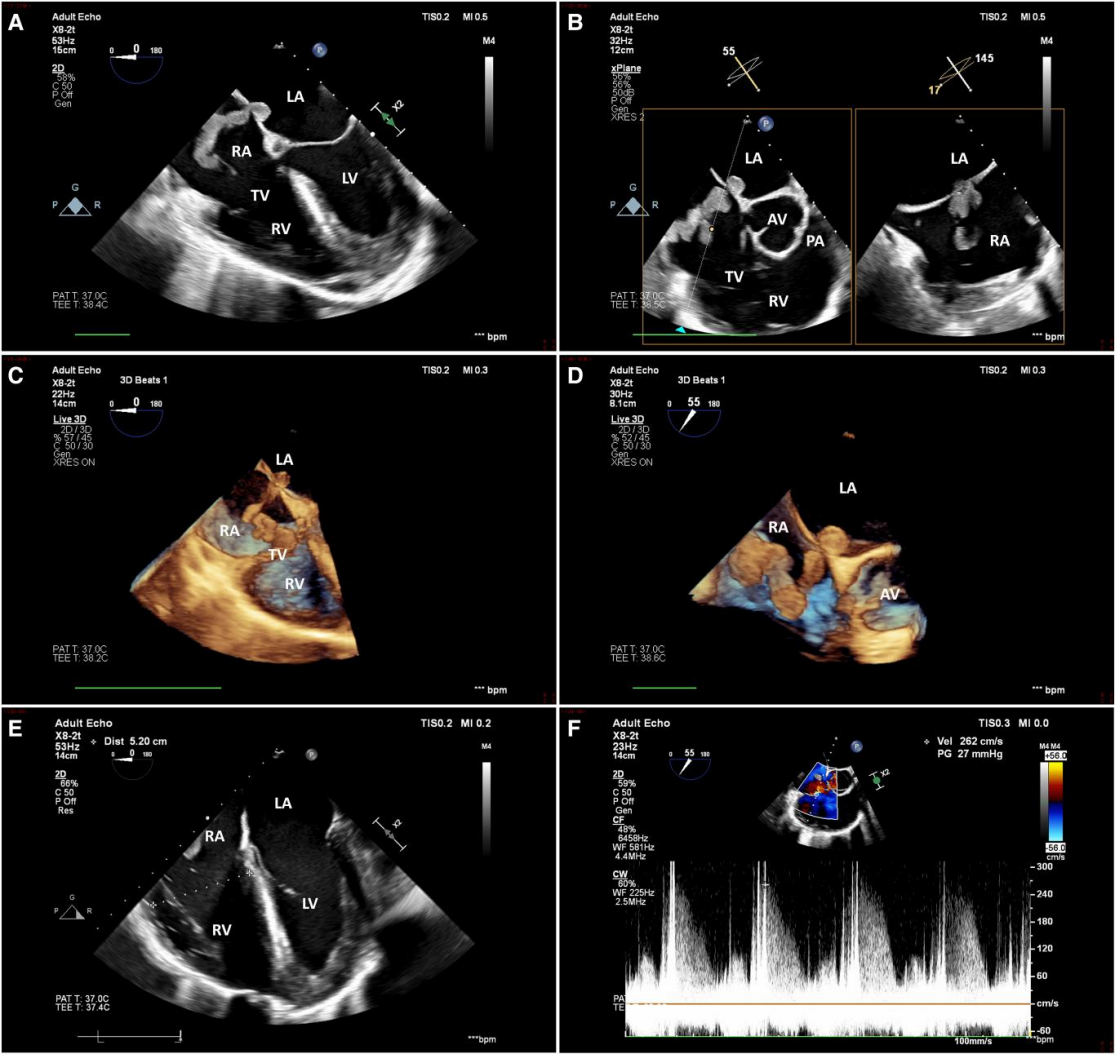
Initial transoesophageal echocardiogram. Initial transoesophageal echocardiogram revealed a large, sausage-like mass lodged in a patent foramen ovale that extended through the right atrium and intermittently protruded beyond the tricuspid valve and into a dilated, dysfunctional right ventricle. A mild-to-moderate tricuspid regurgitation with a peak pressure gradient of 27 mmHg were also demonstrated. *(A* and *E*) Oesophageal 4-chamber view, 2D; (*B* and *F*) oesophageal short axis view, biplane, 2D; (*C*) oesophageal 4-chamber view, live 3D; (*D*) oesophageal short axis view, live 3D. AV, aortic valve; LA, left atrium; LV, left ventricle; PA, pulmonic artery; RA, right atrium; RV, right ventricle; TV, tricuspid valve.
